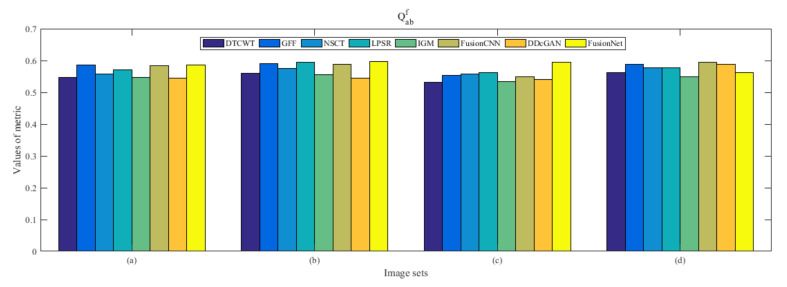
Values of $Q_{ab}^{f}$ in the fused images (MRI-CBF): (a) is composed of eight images in Figure 42, (b) is composed of eight images in Figure 43, (c) is composed of eight images in Figure 44, (d) is composed of eight images in Figure 45.
